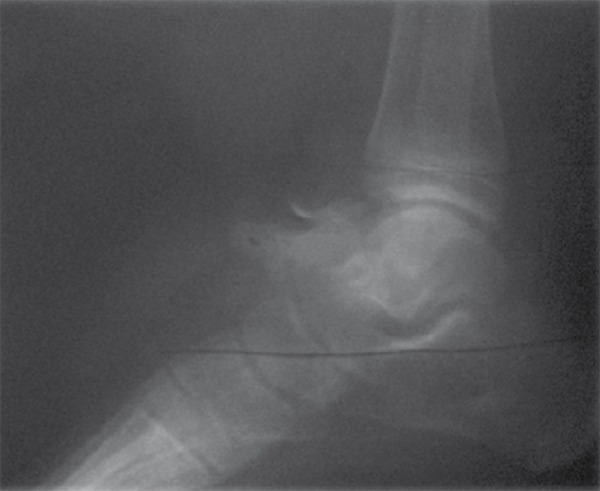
A 17-Year-Old Man Presenting With Foot Pain. Plain film shows subperiosteal osteoid osteoma of the talus which often presents as a round soft tissue mass adjacent to bone.
